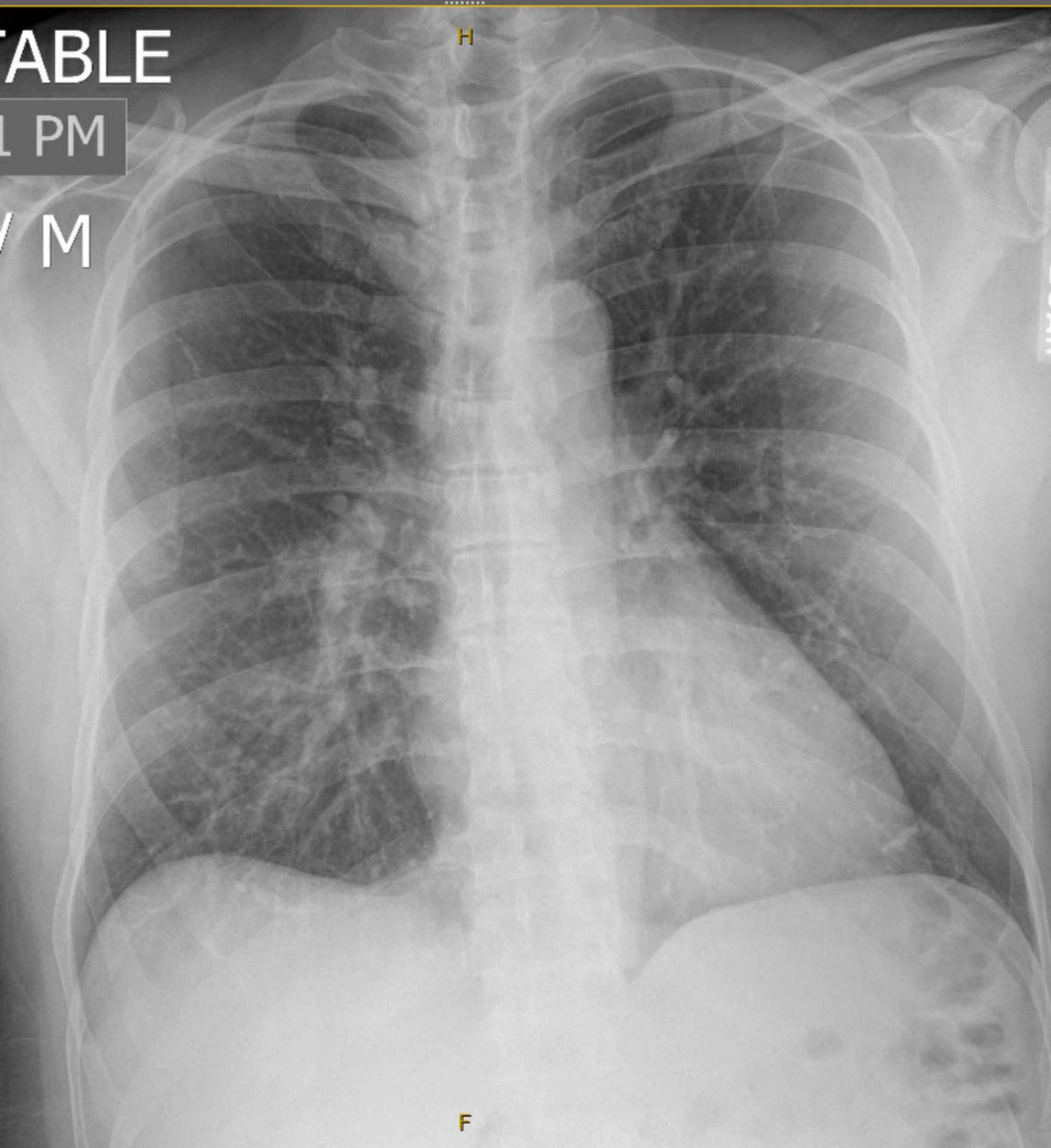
CXR on admission showed no acute cardiopulmonary process. CXR, chest X-ray
